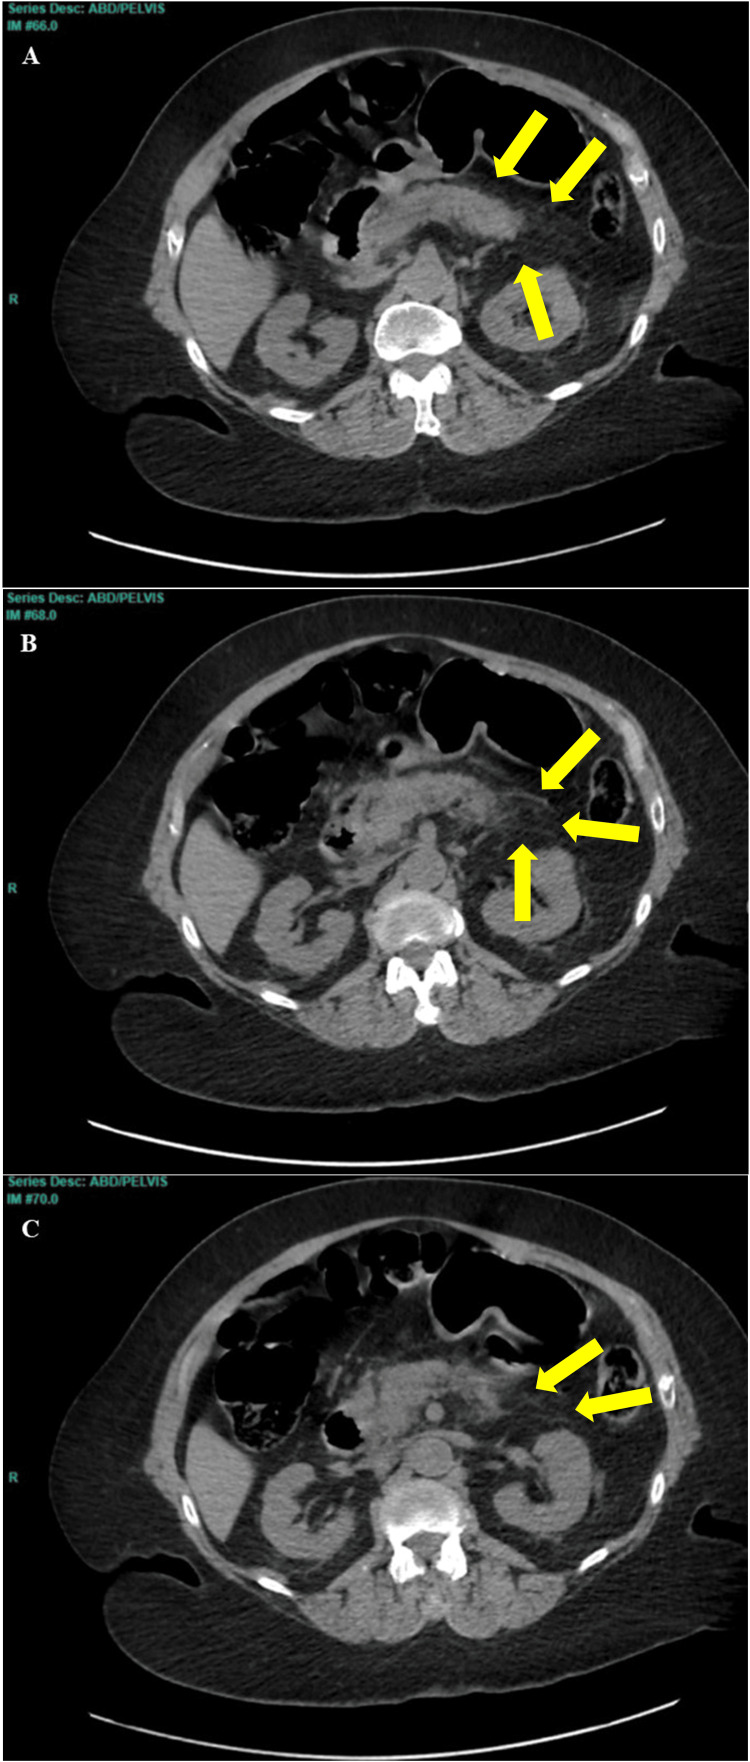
(A-C) Computed tomography of the abdomen and pelvis without intravenous contrast The images show acute interstitial pancreatitis with peripancreatic inflammatory stranding (yellow arrows) and no focal fluid collection. The images are presented in a superior-to-inferior orientation: (A) most superior and (C) most inferior.
